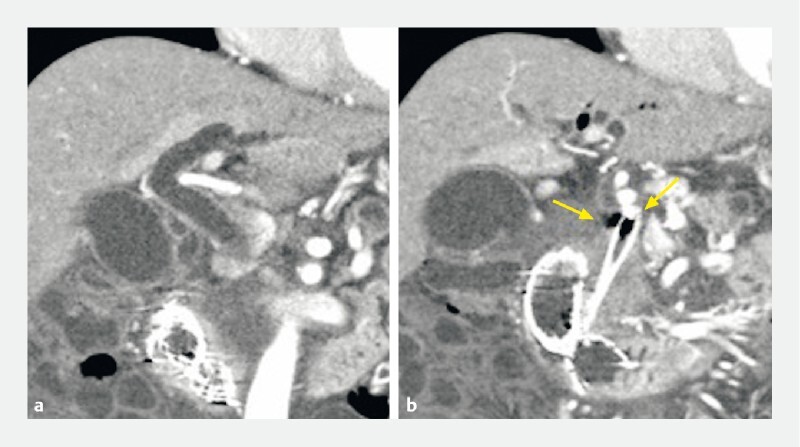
Computed tomography showed bile duct dilation and kinking of a biliary metal stent (arrows).
